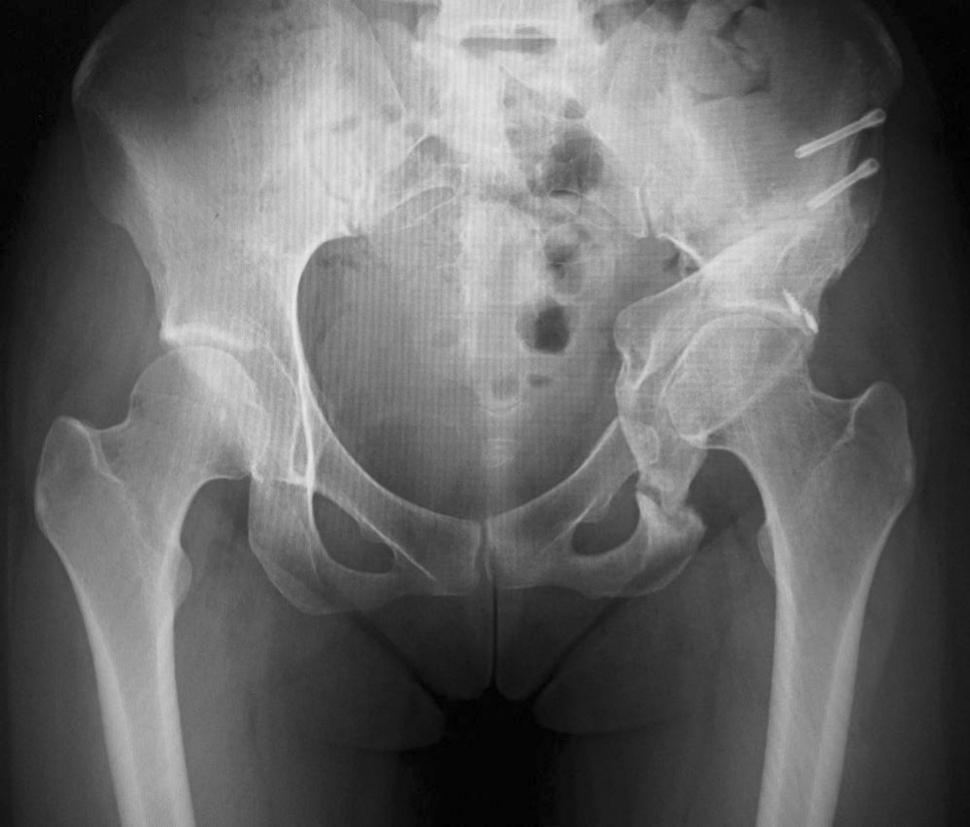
The post-operative anterior–posterior radiograph showed improved acetabular coverage with disappearance of the crossover sign
